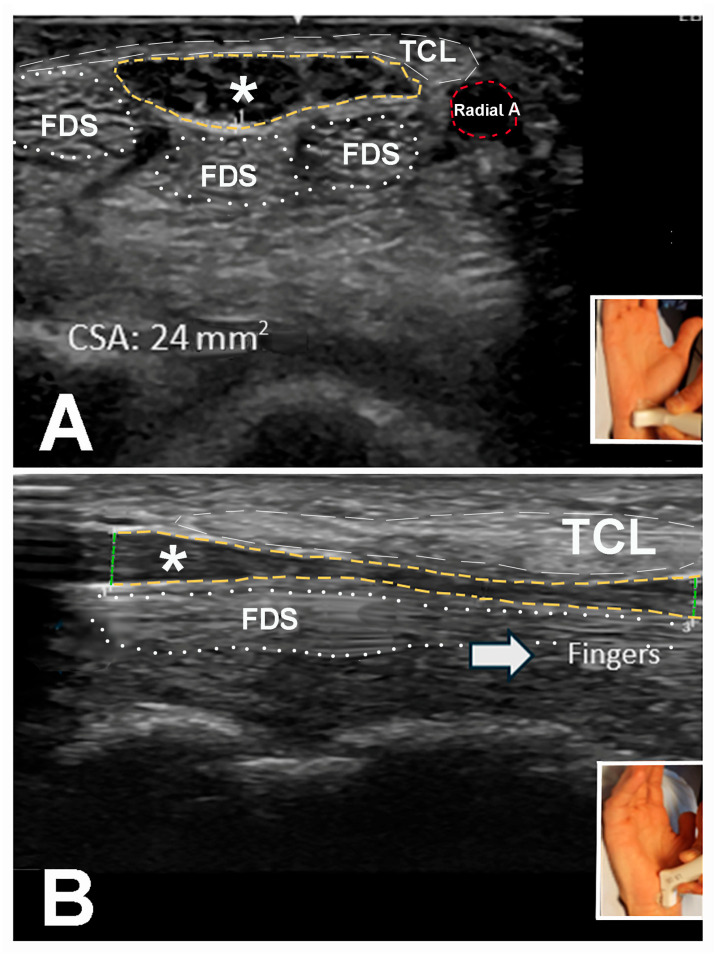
(A) Short-axis view of the median nerve at the carpal tunnel inlet with a cross-sectional area of 24 mm^2^ (normal: 10 mm^2^ or less). (B) Long-axis view of the median nerve within the carpal tunnel showing a decrease in the diameter of the median nerve within the carpal tunnel. The maximum/minimum diameter ratio is calculated from the maximum and the minimum diameter of the median nerve within the carpal tunnel. The insets indicate the positioning of the ultrasound probe for the long-axis and short-axis images of the median nerve. A single asterisk represents the median nerve. The green vertical line to the left of the asterisk in the long-axis view indicates the carpal tunnel inlet. The green vertical line to the right of the asterisk depicts the carpal tunnel outlet. Radial A: radial artery. TCL: transverse carpal ligament. FDS: flexor digitorum superficialis.
